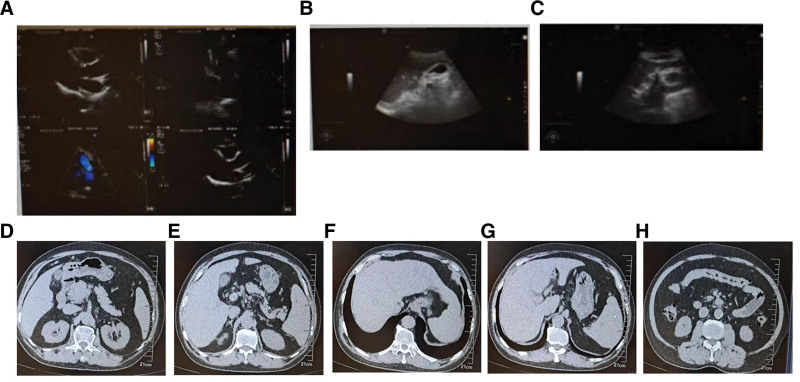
Changes in echocardiography, abdominal ultrasound, and abdominal MRI at admission. (A) Echocardiography; (B,C) abdominal ultrasound; (D–H) abdominal MRI. MRI = magnetic resonance imaging.
